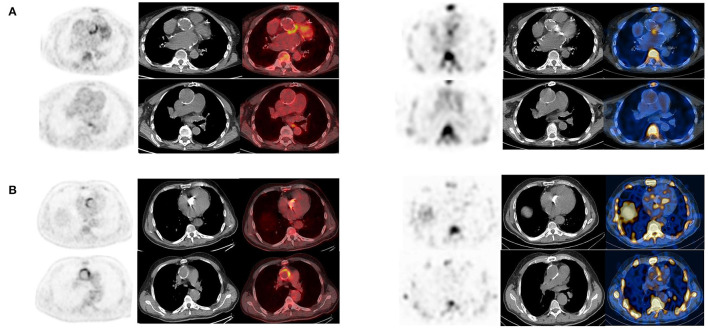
(A,B) Examples of concordant findings between [^18^F]FDG PET/CT (left panel, from left to right transaxial emission, CT and superimposed images) and WBC scan (right panel, from left to right transaxial emission, CT and superimposed images) in two patients with very early infection showing uptake of the corresponding radiopharmaceutical at both the imaging modalities localized at the TG (A) and at both the AV and the TG (B).
